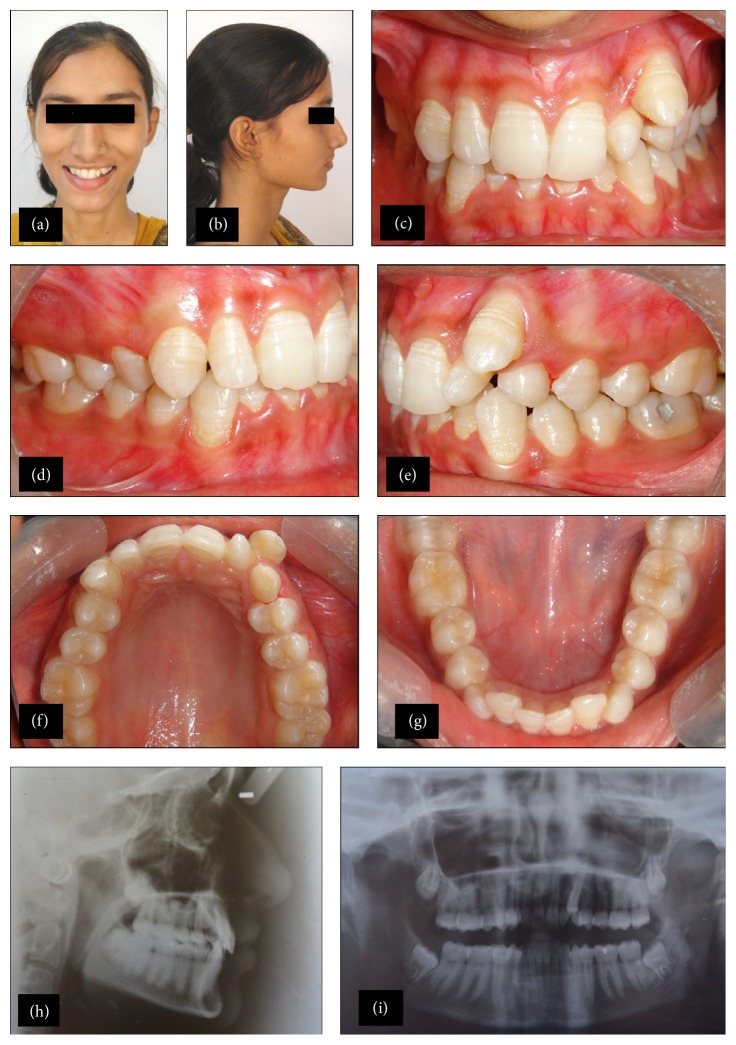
(a) Pretreatment frontal photograph. (b) Pretreatment profile photograph. (c) Pretreatment intraoral frontal view. (d) Pretreatment intraoral right lateral view. (e) Pretreatment intraoral left lateral view. (f) Pretreatment intraoral occlusal view of maxillary arch. (g) Pretreatment intraoral occlusal view of mandibular arch. (h) Pretreatment lateral cephalogram radiograph. (i) Pretreatment panoramic radiograph.
